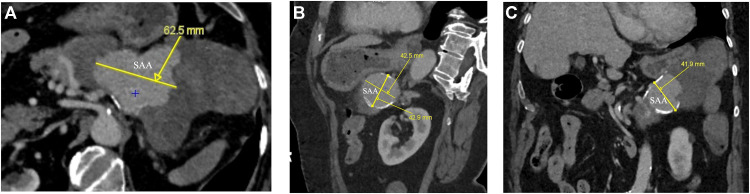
(A) Contrast-enhanced computed tomography (CT), axial multiplanar reconstruction (MPR), demonstrating a large saccular splenic artery aneurysm (SAA), measuring 62 × 42 × 43 mm. (B) Contrast-enhanced CT, sagittal MPR, demonstrating a large saccular splenic artery aneurysm (SAA), measuring 62 × 42 × 43 mm. (C) Contrast-enhanced CT, coronal MPR, demonstrating a large saccular splenic artery aneurysm (SAA), measuring 62 × 42 × 43. CT, computed tomography; DSA, digital subtraction angiography; MPR, multiplanar reconstruction; SAA, splenic artery aneurysm; SAP, splenic artery pseudoaneurysm.
